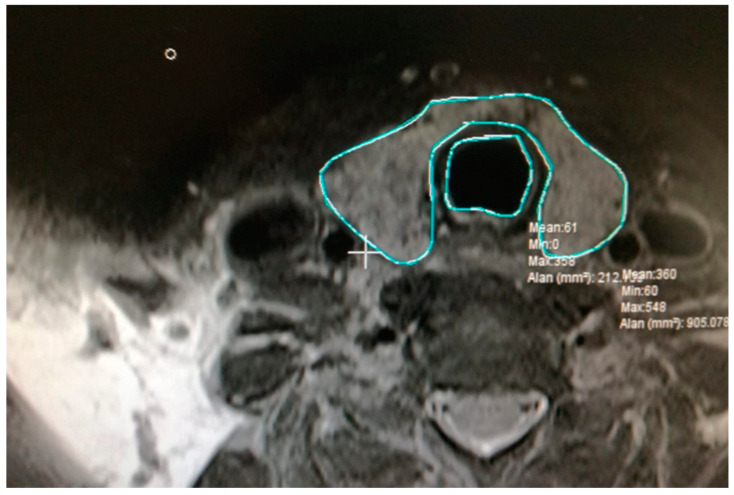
Marked image of the thyroid and trachea outlines in MRI slices.
